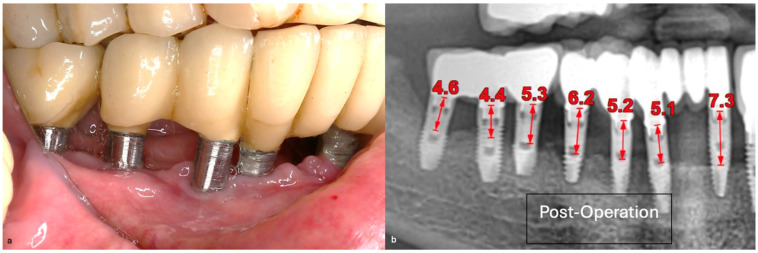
(a) Clinical follow-up after 12 months; (b) Radiographic outcome after 12 months.
